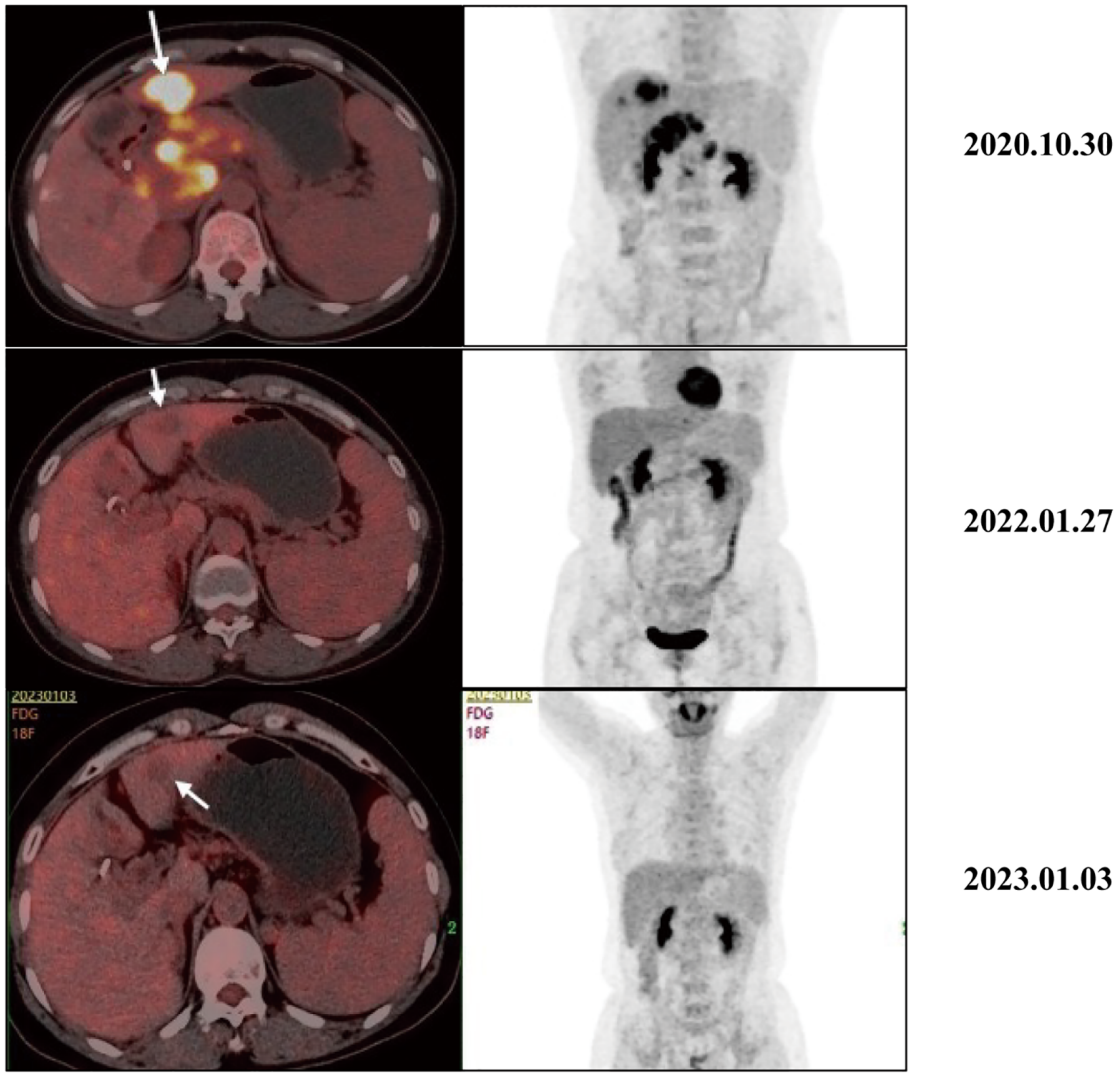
Typical PET-CT images at different time points before and after receiving treatment. The PET-CT of 2020.10.30 showed a mass with high FDG uptake, suggesting metastasis, liver metastasis, portal vein tumor thrombus, and metastasis to peripancreatic and retroperitoneal lymph nodes. After receiving treatment, intrahepatic lesions initially showed a significant decrease in size and FDG metabolism. In the 2022.01.27 and 2023.01.03 images, no increase in FDG metabolism was observed, and the primary peripancreatic and retroperitoneal metastatic lymph nodes and portal vein cancer thrombi have subsided.
